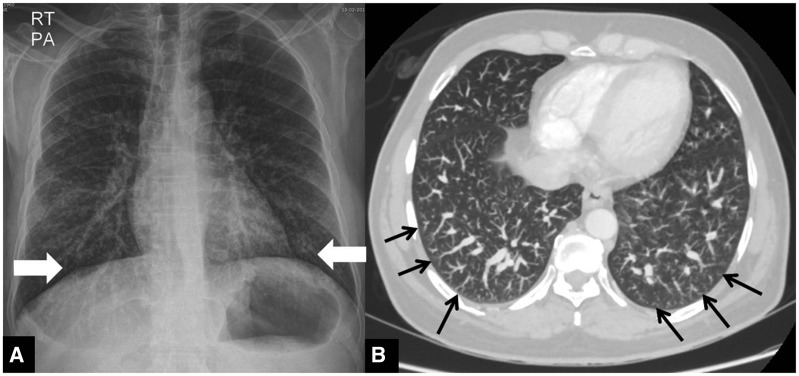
(A) Hepatopulmonary syndrome. Chest radiograph of a case of type 1 hepatopulmonary syndrome in a man with liver cirrhosis showing increased bronchovascular markings in bilateral lower zones (arrows). (B) CT coronal maximum intensity projection image of lung window showing dilated distal pulmonary arteries with subpleural telangiectasia (arrowheads).
